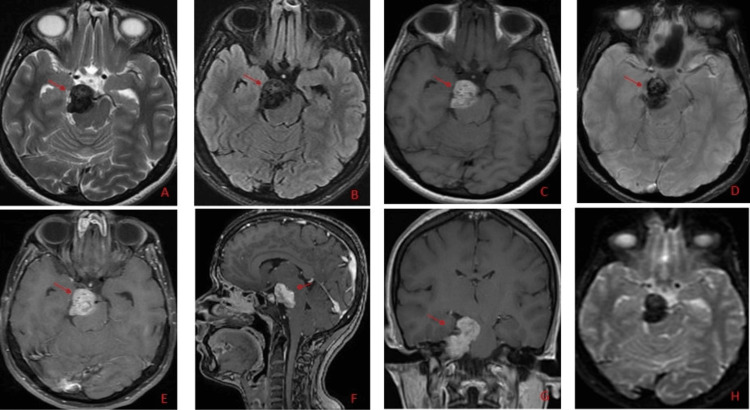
Pre-operative MRI brain showed a well-defined extra-axial lobulated mass (red arrows) measuring 3.7 × 3.6 × 2.1 cm mass in the right trigeminal cisterna (T2 axial image - A, FLAIR axial image - B), post-contrast T1 fat sat images - (sagittal image F and coronal image G) with extension into Meckel’s cave. The tumor encased the right posterior cerebral artery causing a mass effect on the adjacent structures (image E). There was no extension in the internal auditory canal. There was no diffusion restriction (H). It appeared hyperintense on T1-weighted images (C) and hypointense on GRE images (D), suggestive of a bleed FLAIR: Fluid-attenuated inversion recovery
